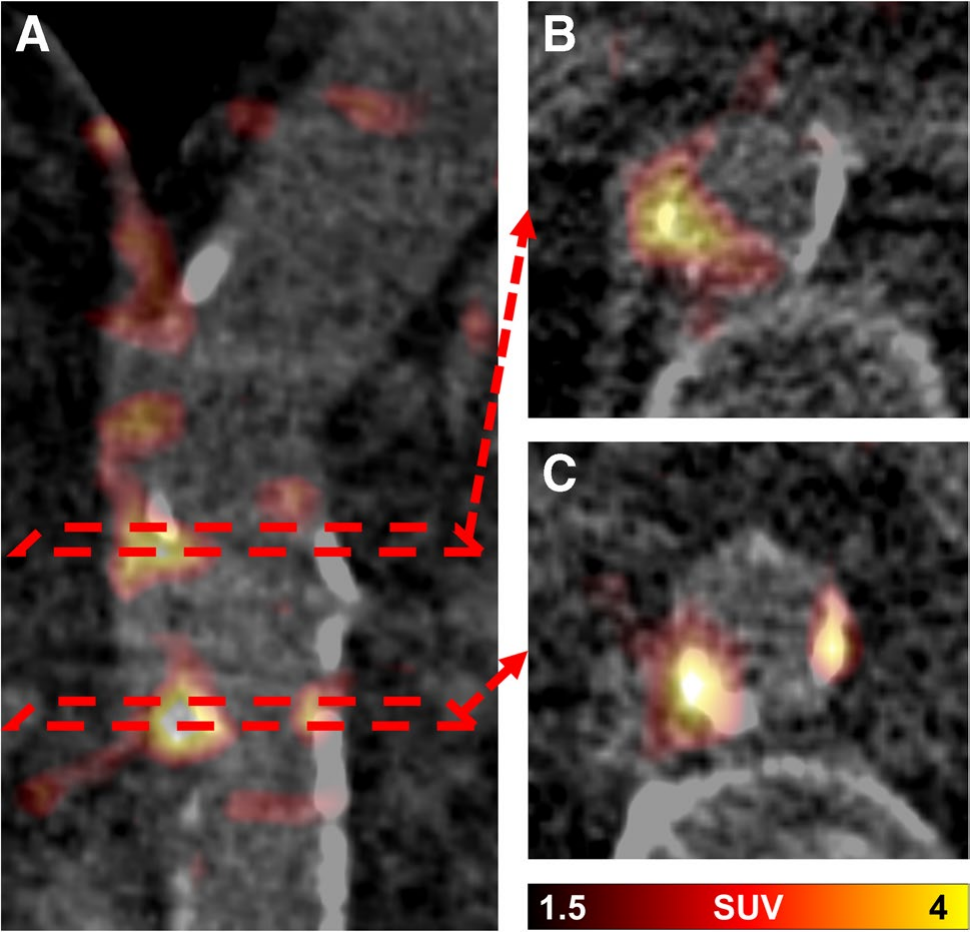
Fused axial [^68^ Ga]Ga fibroblast activation protein inhibitor (FAPI) PET/CT images of the aorta of a 72-year-old man: coronal PET/CT image (A) and corresponding axial PET/CT slices (B and C). In B, radiotracer uptake in the vessel wall coincides with and exceeds vessel wall calcification, while other calcifications show no increased tracer uptake. In C, FAPI uptake is colocalized with vessel wall calcifications
